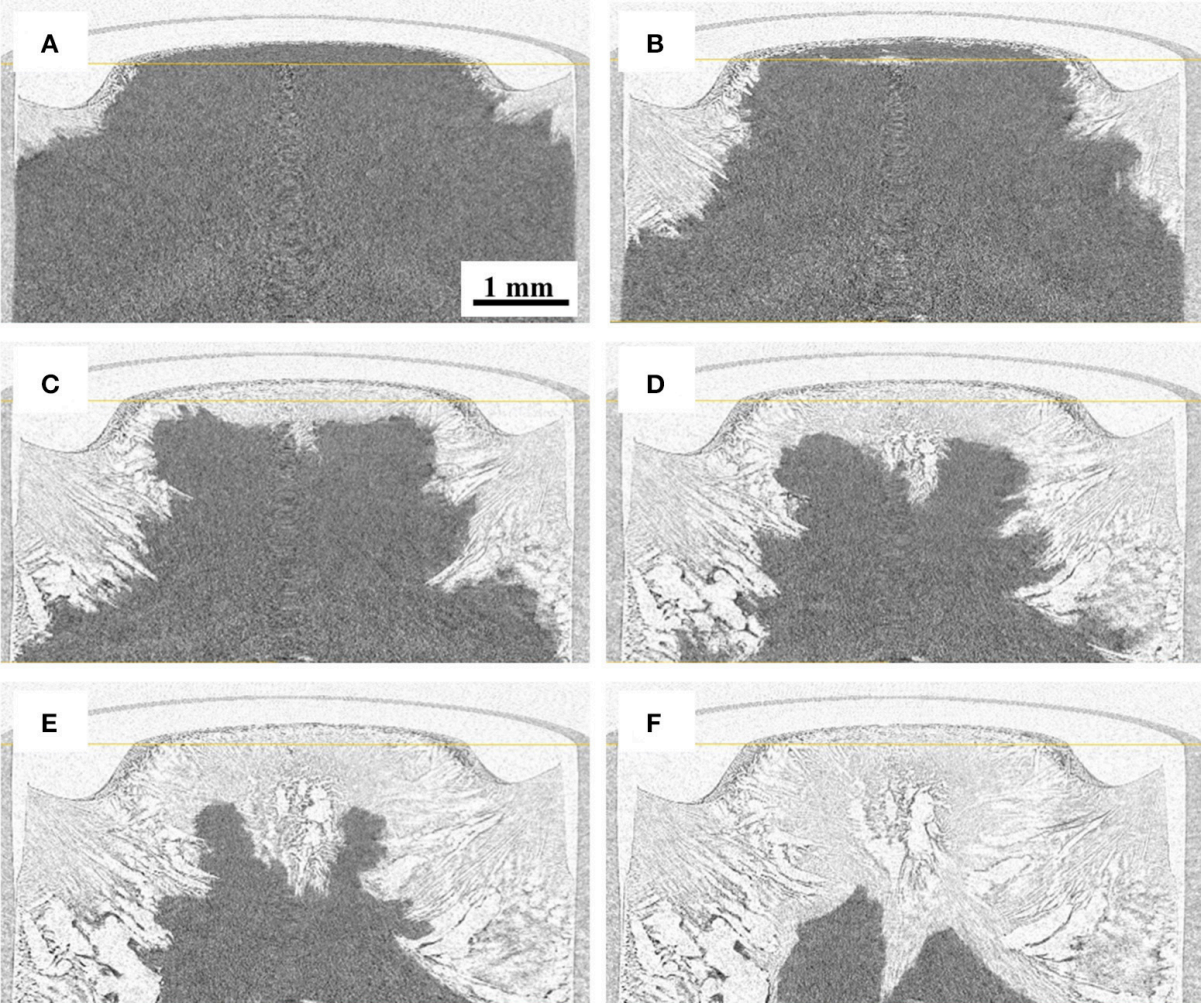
Three-dimensional (3D) X-ray CT images during freeze-drying of the non-annealed samples, dried for (A) 600, (B) 1,200, (C) 1,800, (D) 2,400, (E) 3,000, and (F) 3,900 s.
